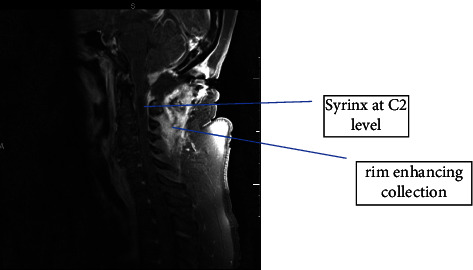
Sagittal section of MRI brain with/without contrast showing a rim-enhancing collection and 2.3 cm × 0.9 cm syrinx at the level of C2.
